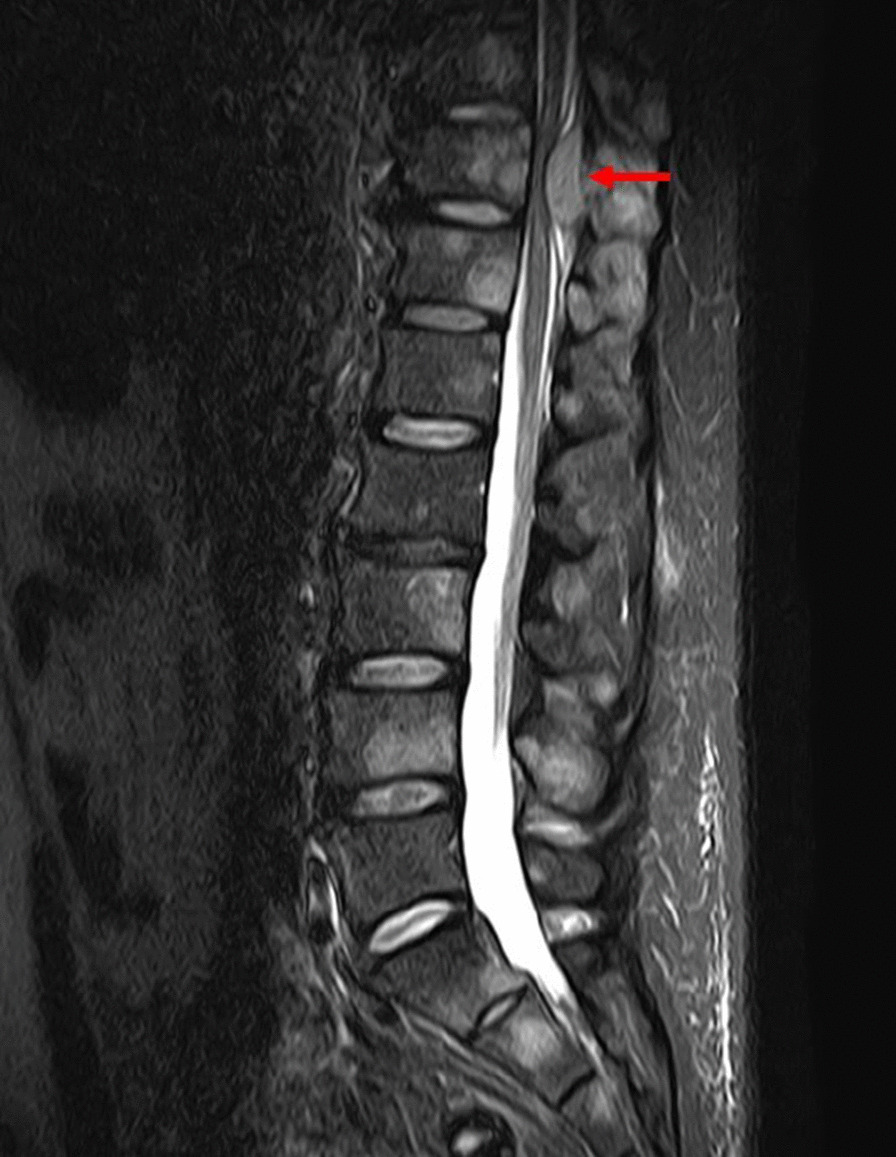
Magnetic resonance imaging of the lumbar spine showing an epidural mass at the level of thoracic vertebra 11 (Th11; red arrow) and multiple high-intensity vertebral lesions on short-time inversion recovery imaging
